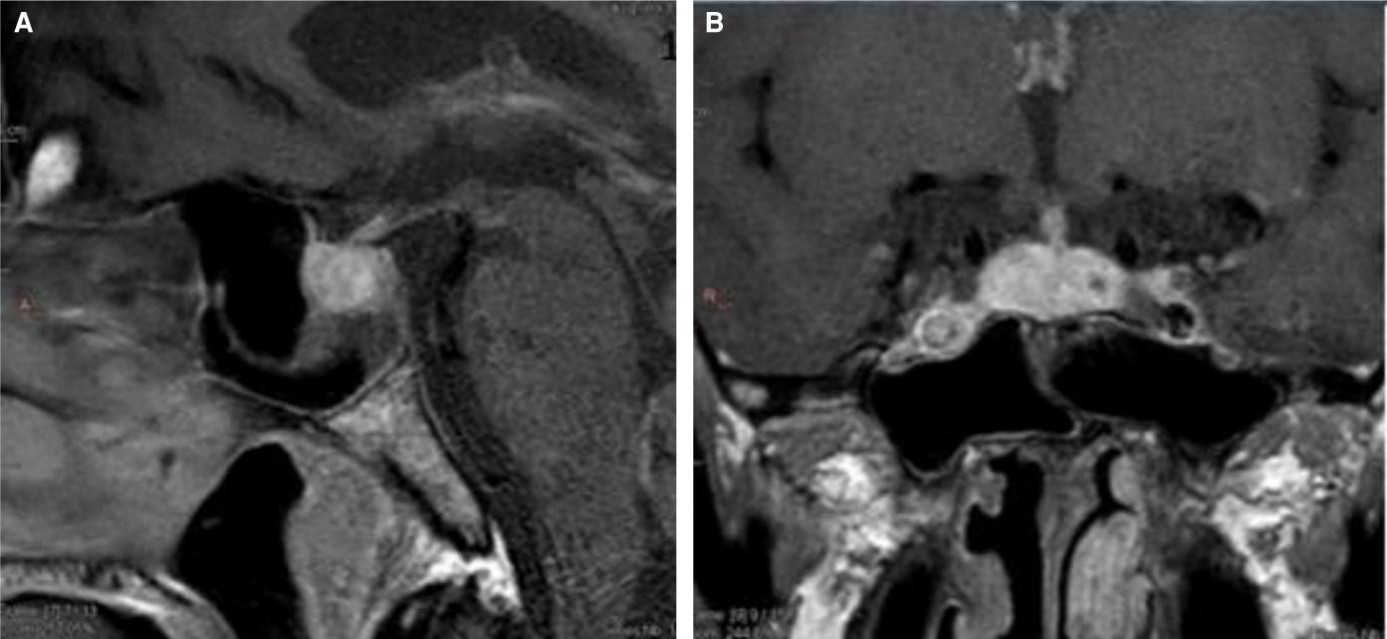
T1-weighted pituitary magnetic resonance imaging. (A) (sagittal view) and (B) (frontal view), taken at hypophysitis diagnosis, show global enlargement of the pituitary gland and heterogeneous enhancement after gadolinium injection.
